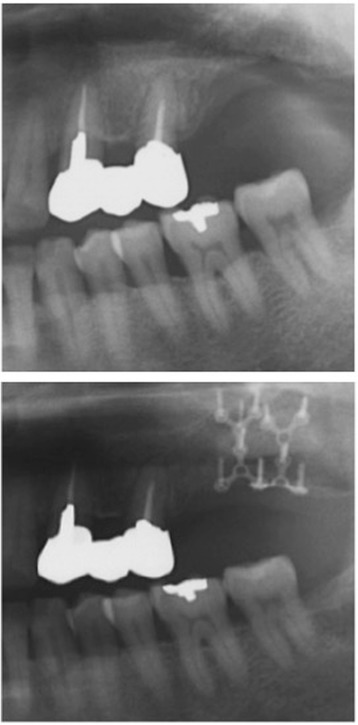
Preoperative and postoperative radiograph
